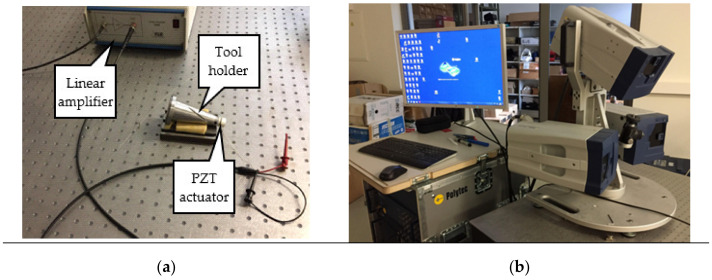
Actual experimental set-up of the vibration response of the tool holder with and without helical slots. (a)—view of the tool holder with a fixed piezoelectric actuator and a linear amplifier P200 (FLC Electronics AB, Partille, Sweden), (b)—a view of the Polytec PSV-500 3D laser doppler vibrometer-scanner (Polytec GmbH, Widbronn, Germany).
